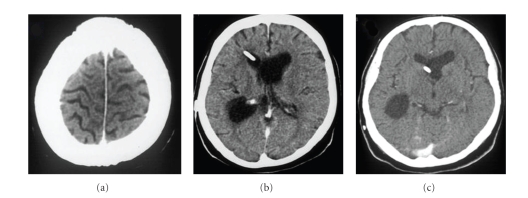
Noncontrast CT scans after endoscopic intraventricular cystic drainage and repositioning of the ventricular shunt (2006).
